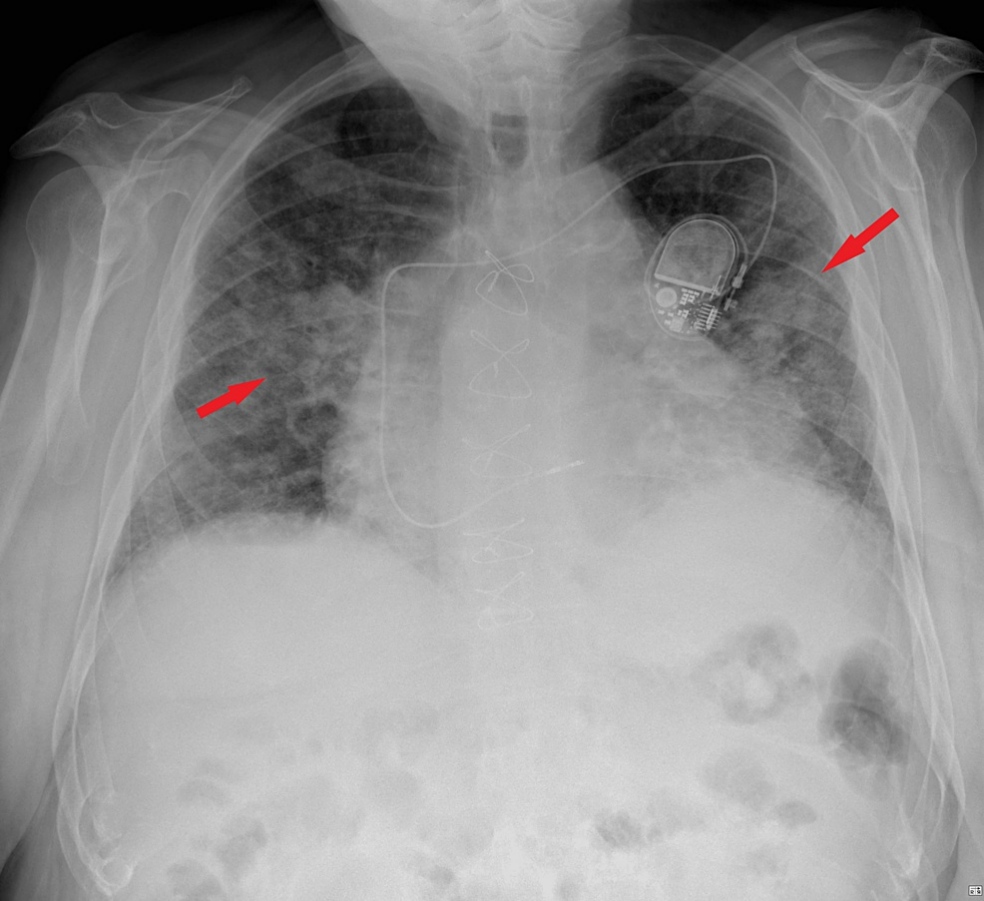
Chest x-ray during acute phase showing diffuse bilateral, infiltrative opacification patterns, sparing the pulmonary apex consistent with an acute diffuse alveolar hemorrhage (represented by the red arrows)
